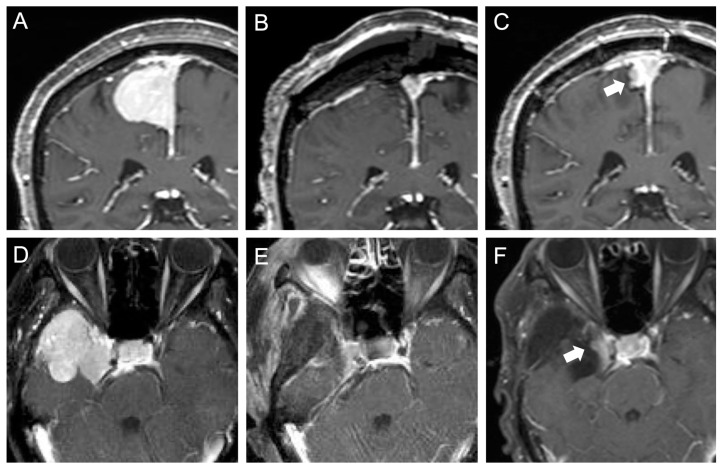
Demonstrative cases of a non-skull base (A–C) and a skull base (D–F) meningioma with dural sinus involvement showing early recurrence after gross total resection. (A) Preoperative T1Gd coronal image shows parasagittal meningioma involving superior sagittal sinus. (B) Early postoperative MRI on POD1 shows no apparent residuals. (C) Follow-up MRI 6 months after surgery shows the recurrent tumor adjacent to the superior sagittal sinus (arrow). The patient was closely followed with repeated MRI in another 6 months, which showed further growth of the recurrent tumor. Stereotactic radiation therapy was administered and the tumor regressed without any regrowth for 3 years. (D) Preoperative T1 Gd axial image shows right medial sphenoidal ridge meningioma involving cavernous sinus. (E) Early postoperative MRI on POD1 shows no apparent residuals. (F) Follow-up MRI 7.5 months after surgery shows the recurrent tumor at the lateral wall of the cavernous sinus (arrow). Gamma knife surgery was performed and the tumor size was stable for 4.5 years, after which it showed regrowth and required surgical resection.
